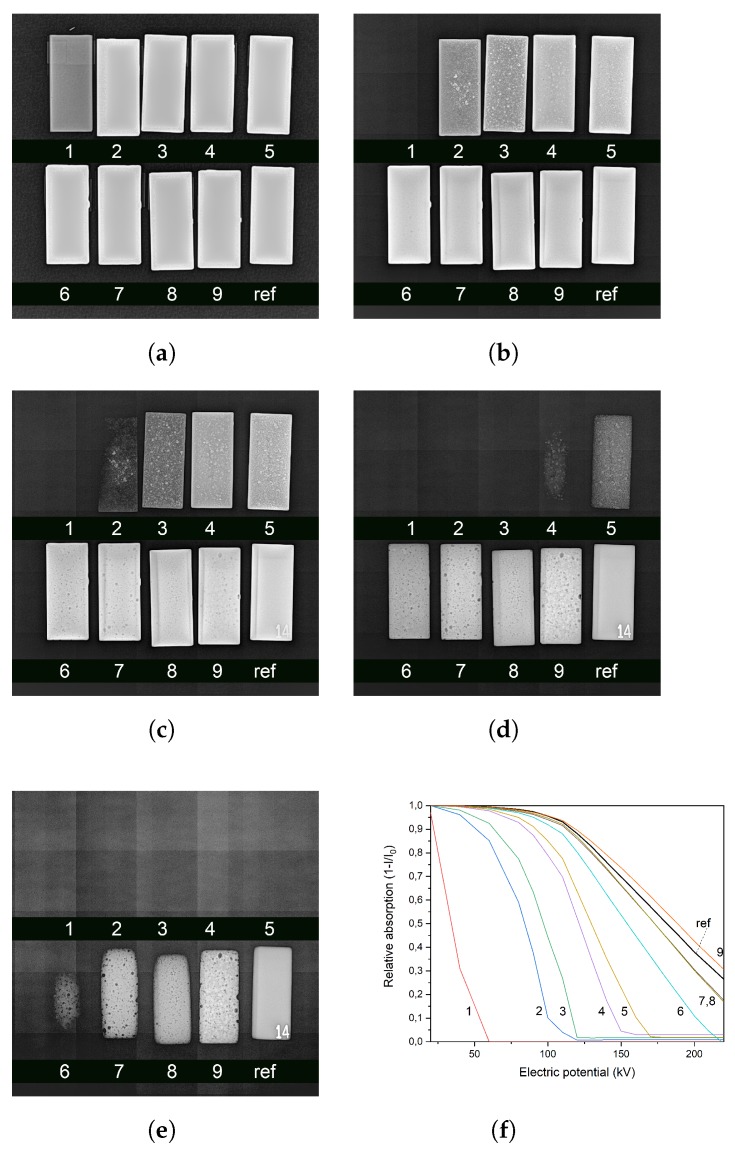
Radiographies of epoxy-barite samples at increasing percentage *w*/*w* in BaSO_4_. Samples are labeled as in Table 2. Ref sample is the reference of steel. In (f), increasing X-ray absorbance profiles can be osberved, from left (0% *w*/*w* barite) to right (85% *w*/*w* barite). Greyscale data associated to (f) and relative statistics are available as supplementary material. (a) 20 kV; (b) 60 kV; (c) 100 kV; (d) 150 kV; (e) 220 kV; (f) Profile comparison.
